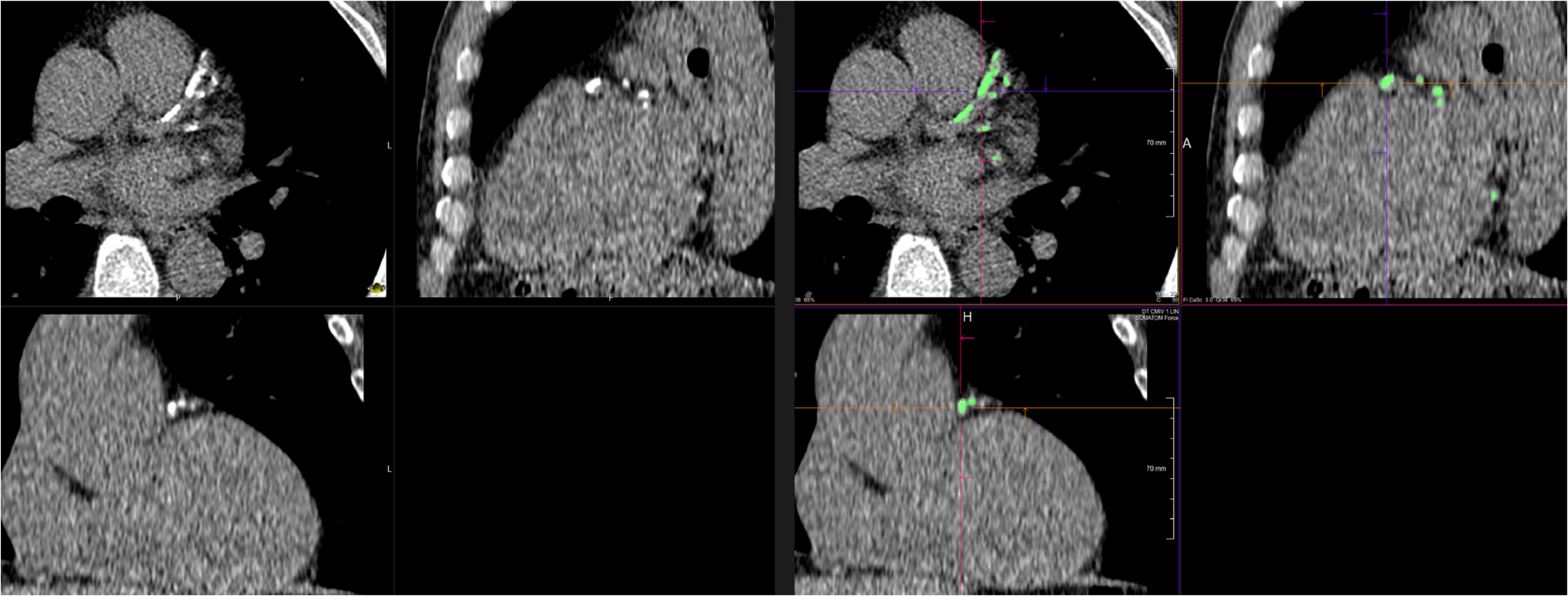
Multiplanar reconstructions of a coronary calcium scoring computed tomography scan with extensive calcifications in left anterior descending artery and circumflex artery. Left: Pre-processing images demonstrating calcified lesions as high attenuation regions. Right: Post-processing, visual coronary calcium scoring feedback. High attenuation regions that contributed to the results are displayed in green color
